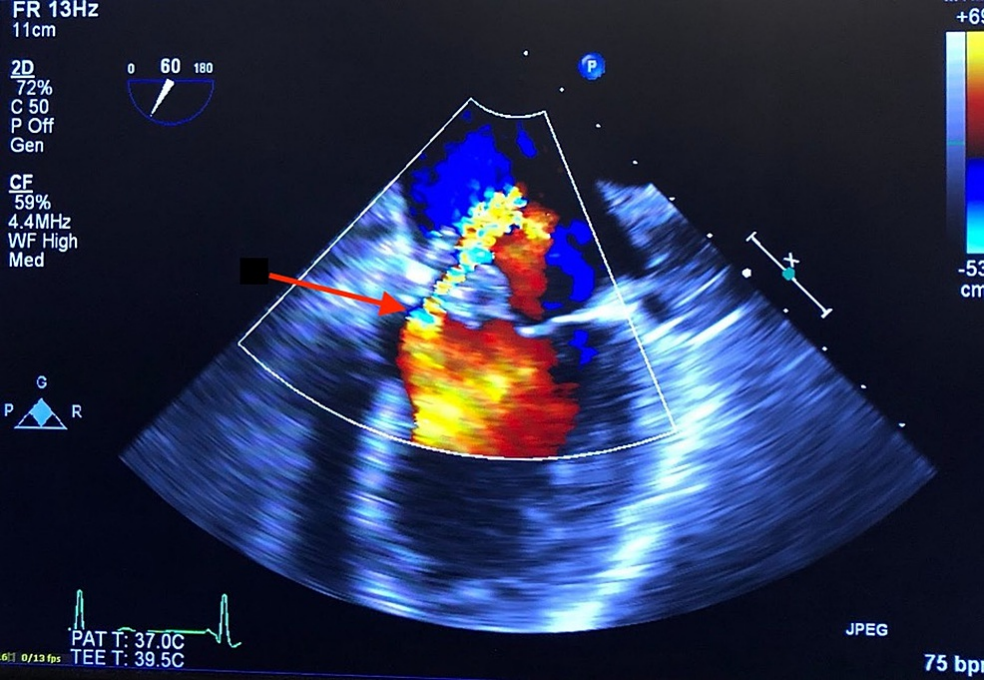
Transesophageal echocardiogram with a mid-position view showing severe mitral regurgitation (red arrow) going through the necrotic core of the vegetation
